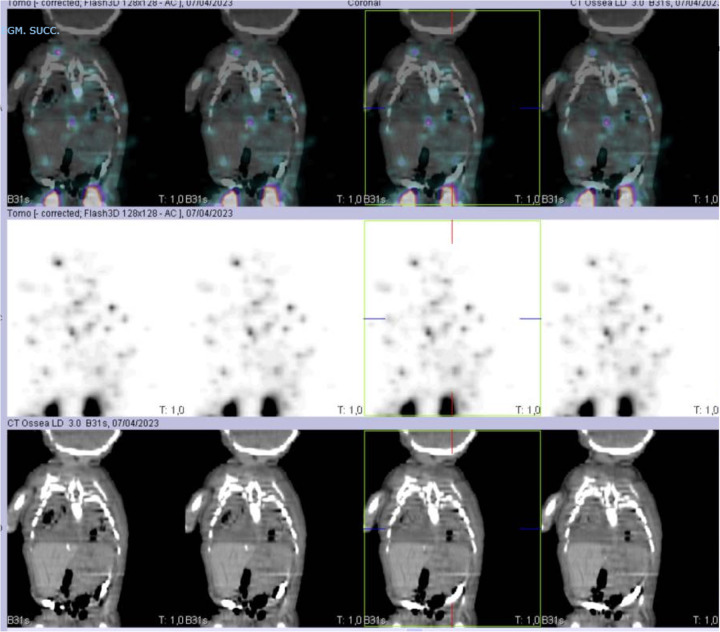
Lymphoscintigraphy performed at approximately 3 months of life. Bilateral transit of the radiopharmaceutical tracer was documented up to the thoracic duct, visualized in its thoracic portion. A minimal amount of tracer was detected at the level of the known pleural effusion, particularly in the left basal and bilateral apical regions. No pathological tracer accumulation was observed in the abdominal compartment at standard imaging timepoints. However, approximately 20 h after tracer administration, lymphatic fluid was aspirated from the abdominal drainage catheter and analyzed by gamma-counter; two 2 mL samples demonstrated significant radioactivity counts (841 and 791 cpm), indicating that the chylous ascites originated from thoracic lymphatic leakage rather than from an independent abdominal source.
